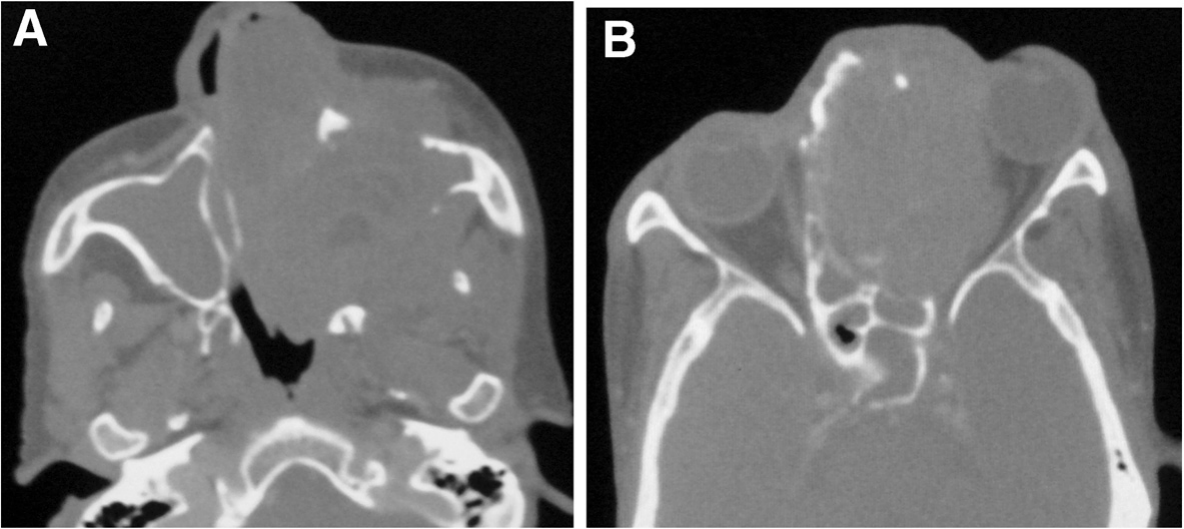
CT images in bone window showing bony destruction. A &B: Axial section CT images (bone window) showing destruction of medial and postero-lateral wall of left maxillary sinus, left lateral wall of nasal cavity, nasal septum, ethmoid sinus and medial wall of left orbit by the mass.
